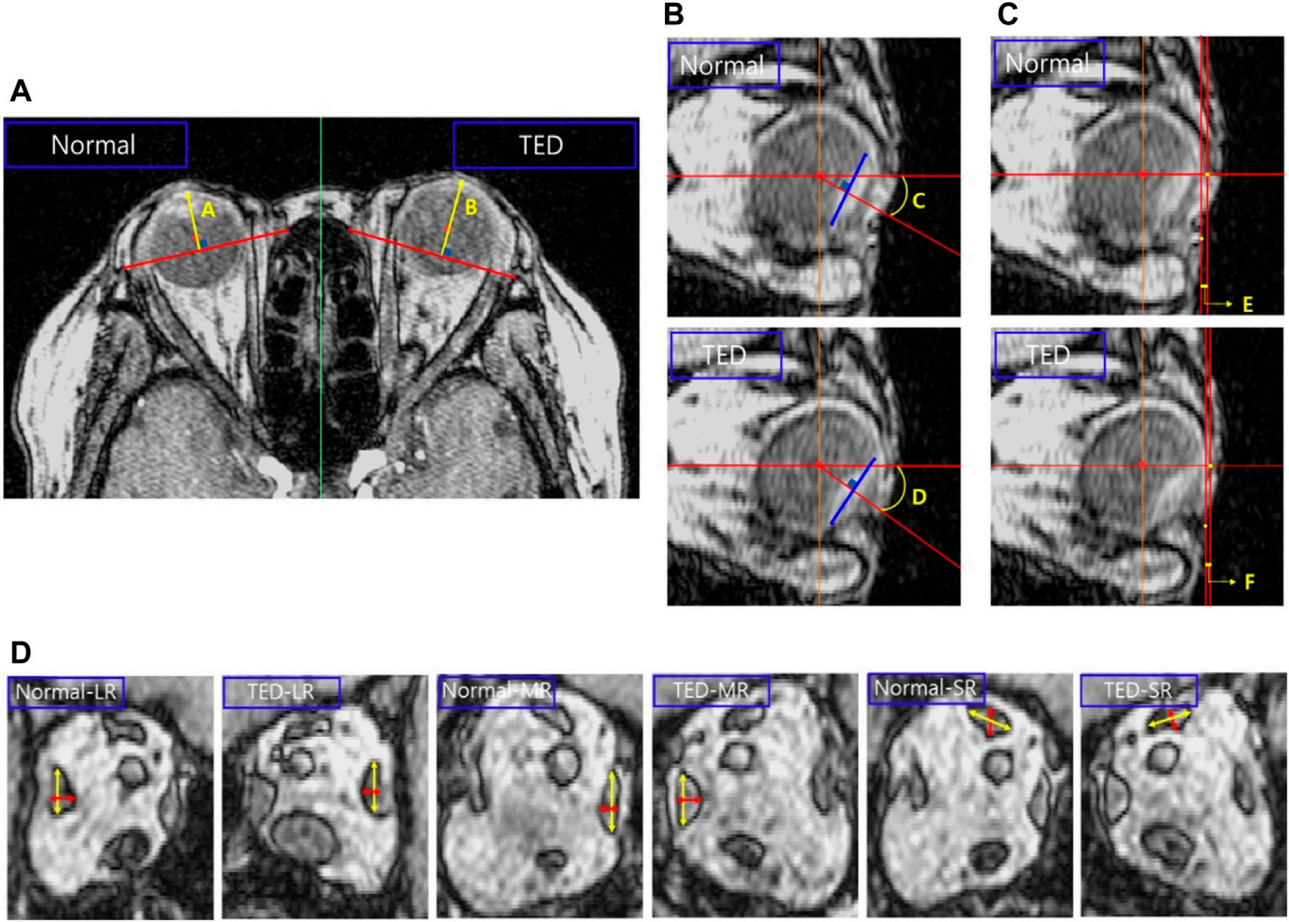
Analysis of major dimensions in the thyroid eye disease (TED) orbit and normal orbit based on magnetic resonance imaging (MRI). (A) Proptosis measurement. (B) Eye rotation measurement. (C) Distance measurement between the ocular and skin end point. (D) Thickness and width measurements in the extraocular muscle (EOM) except for the inferior rectus (IR) muscle.
